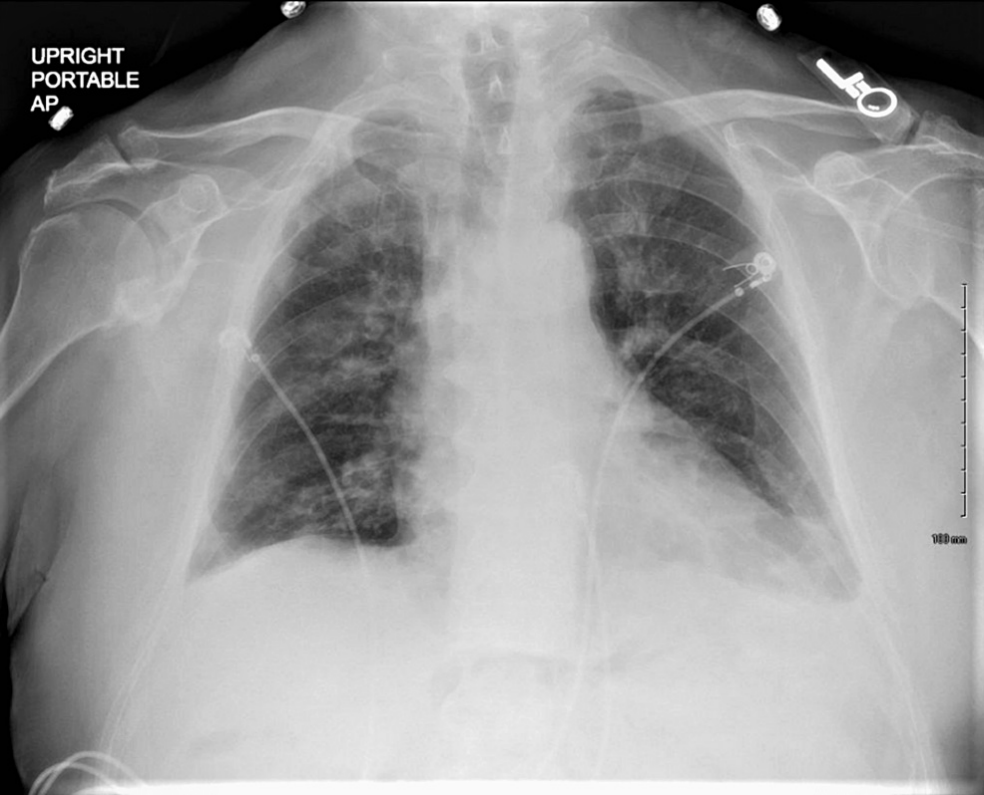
Follow-up chest X-ray showing decreased lung opacities bilaterally
